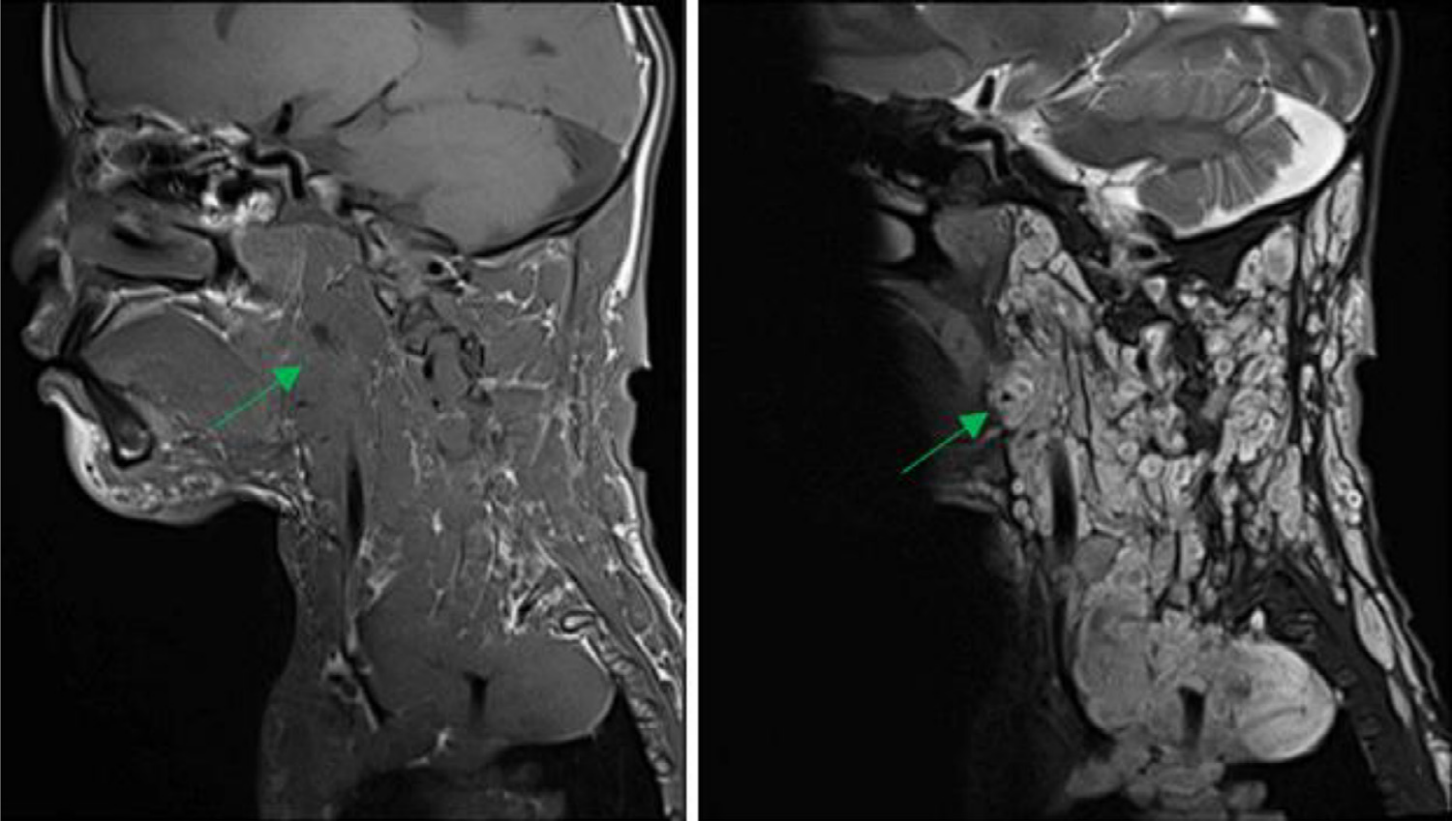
Cervical MRI of the patient with plexiform neurofibroma. (Left) hypointense signal on T1-weighted imaging; (Right) hyperintense signal on T2-weighted imaging.
